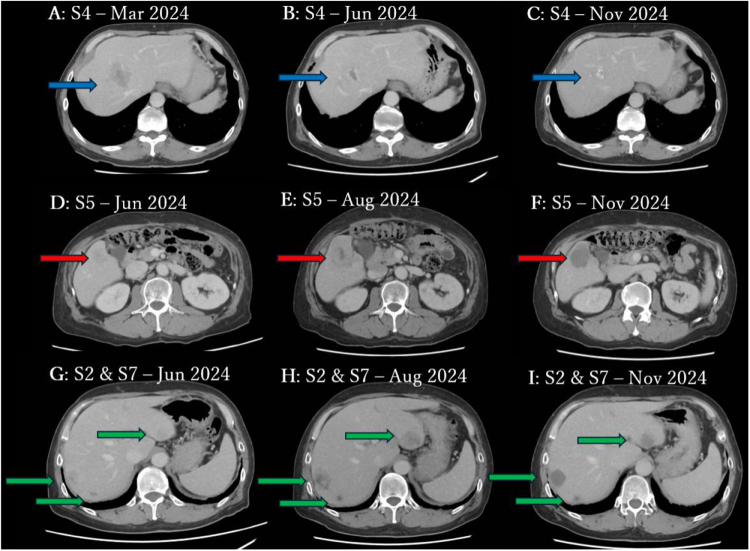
Serial contrast-enhanced CT images demonstrating differential responses of liver metastases to panitumumab- and bevacizumab-based chemotherapy, corresponding to the lesions listed in Table 1. (A–C) Lesion L3 located in liver segment 4 (S4) is shown in March 2024 (A), June 2024 (B), and November 2024 (C) (blue arrows). This lesion showed tumor shrinkage during panitumumab-based therapy, which was further maintained after switching to bevacizumab, accompanied by loss of contrast enhancement. (D–F) Lesion L5 located in segment 5 (S5) is shown in June 2024 (D), August 2024 (E), and November 2024 (F) (red arrows). This lesion progressed with enlargement and persistent contrast enhancement during panitumumab-based therapy, but markedly regressed with disappearance of enhancement after switching to bevacizumab. (G–I) Lesions L1 (segment 2) and L6/L7 (segment 7) are shown in June 2024 (G), August 2024 (H), and November 2024 (I) (green arrows). These lesions similarly demonstrated progression during panitumumab-based therapy, followed by significant regression and loss of contrast enhancement after initiation of bevacizumab. Lesion numbering corresponds to that used in Table 1.
